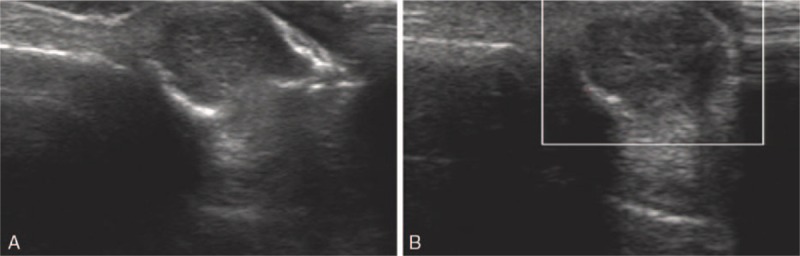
Case 1. (A) Two-dimensional ultrasound showed a hypoechoic mass located in and beyond the medullary cavity of the phalanx. (B) Doppler ultrasound showed no blood signal in the mass.
